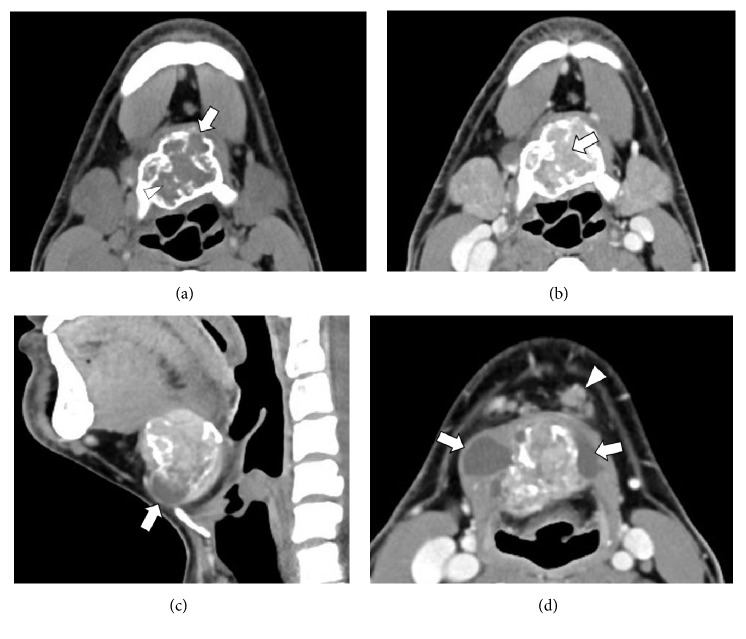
CT of the neck. (a) Axial noncontrast CT at the level of the hyoid bone shows a well-defined, low-density mass expanding the cortex of the hyoid bone with partial bone destruction (arrow). Punctate calcifications are seen within the mass (arrowhead). (b) Axial contrast-enhanced CT at the same level as (a) shows marked enhancement of the solid component (arrow). (c, d) Sagittal at the midline (c) and axial at the infrahyoid level (d) images of contrast-enhanced CT show the mass consists of a solid component and a marginal cystic component (arrows). Left submental lymphadenopathy is also seen (arrowhead).
